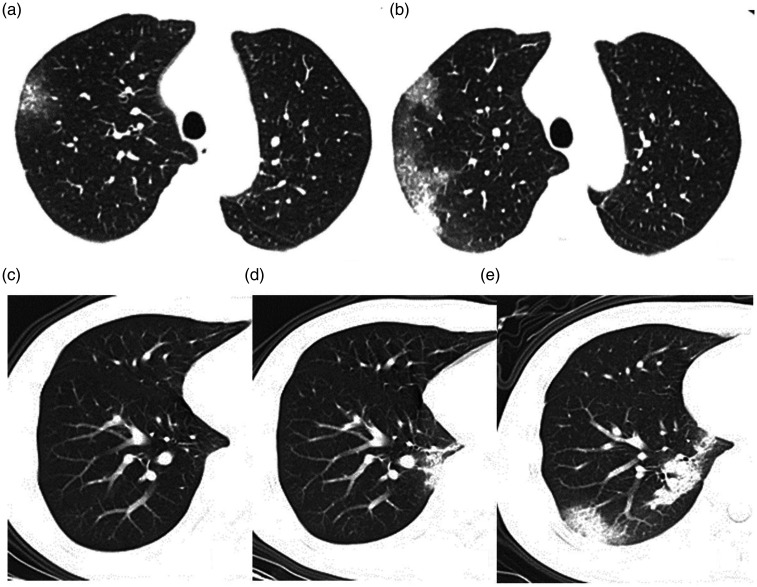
(a, b) Case 10. (a) CT scan two days after onset of symptoms (vascular congestion). (b) CT scan seven days after the onset of symptoms (part consolidation). (c– e) Case 7. (c) CT scan on the day of onset of symptoms (negative on this CT slice), (d) CT scan three days after onset of symptoms (GGO with a reticular parttern), (e) CT scan seven days after onset of symptoms (consolidation). Both cases show the number of lesions, and the extent of the lesions increased as the disease progressed. CT, computed tomography; GGO, ground-glass opacity.
